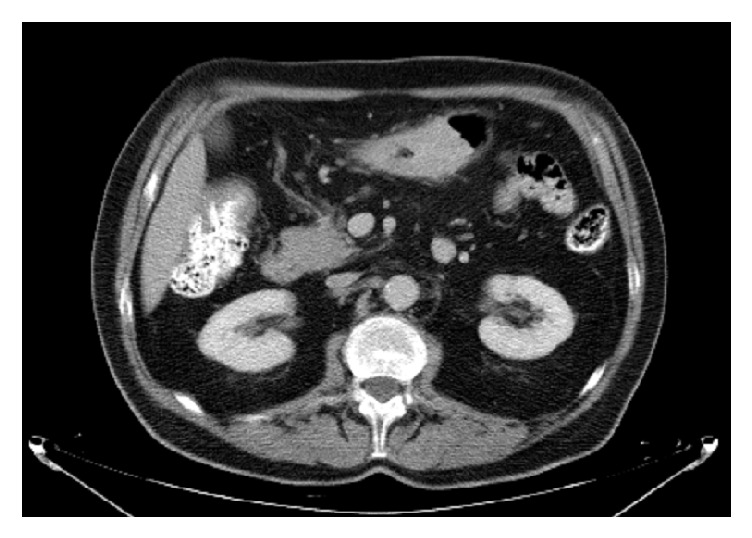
Abdominal CT scan showing stenotic GIP.
